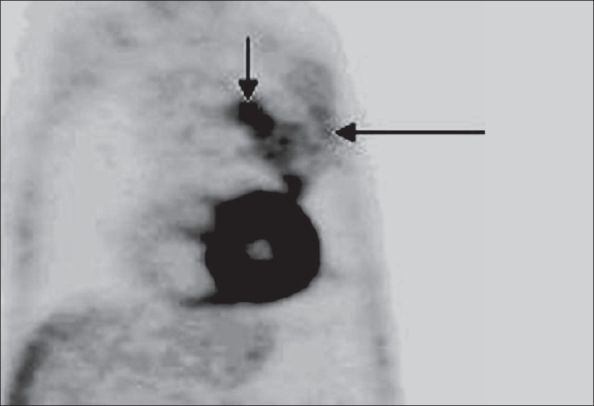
Coronal F18 FDG PET image shows that the peripheral and inferior portions are relatively less metabolically active (long arrow) as compared to the superomedial portion (short arrow), which shows the maximum metabolic activity
